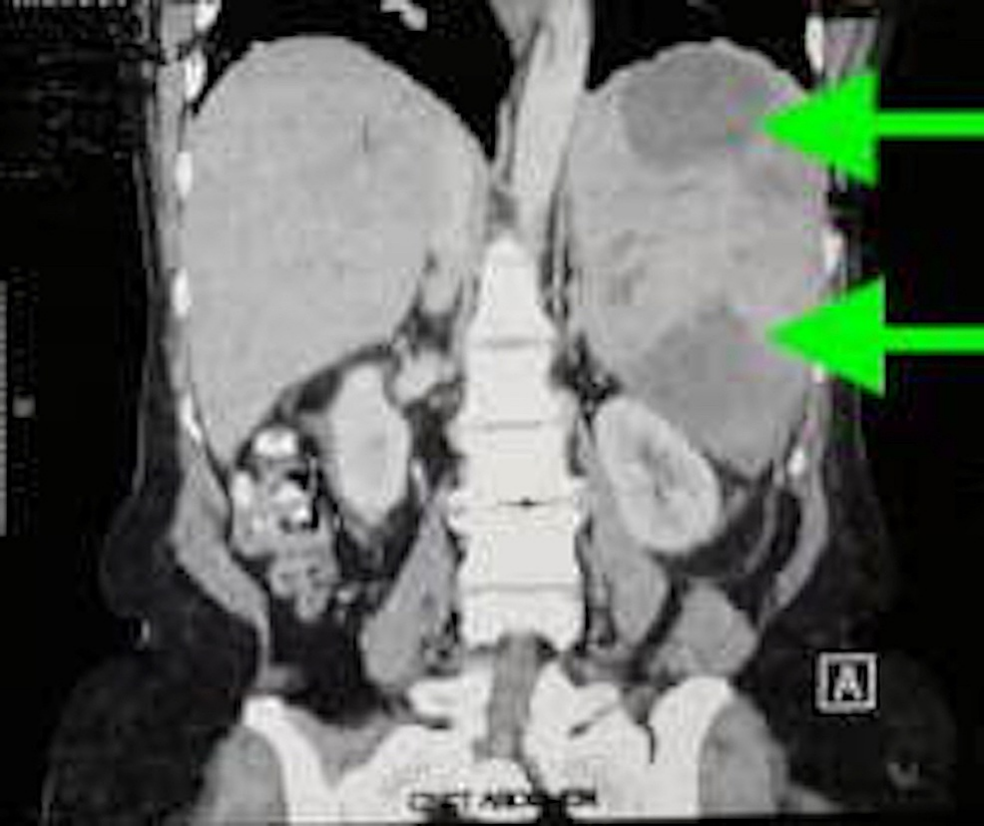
CECT of the abdomen showing hepatosplenomegaly with few irregular hypodense areas in the spleen suggestive of infarction (green arrows). CECT: Contrast-enhanced CT.
